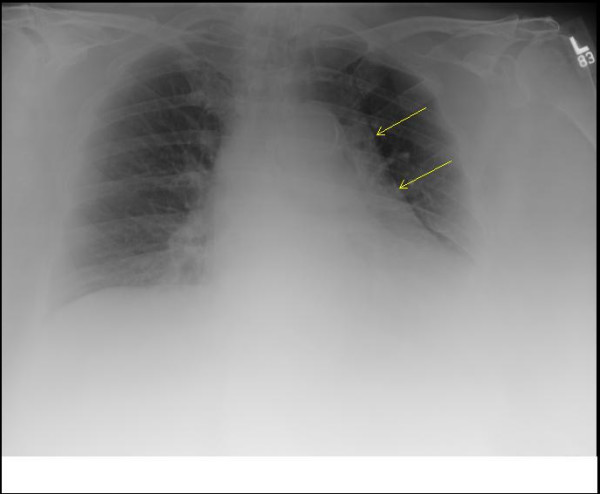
Chest radiograph showing presence of mediastinal widening with a paracardiac shadow of increased lucency giving a double contour effect.
